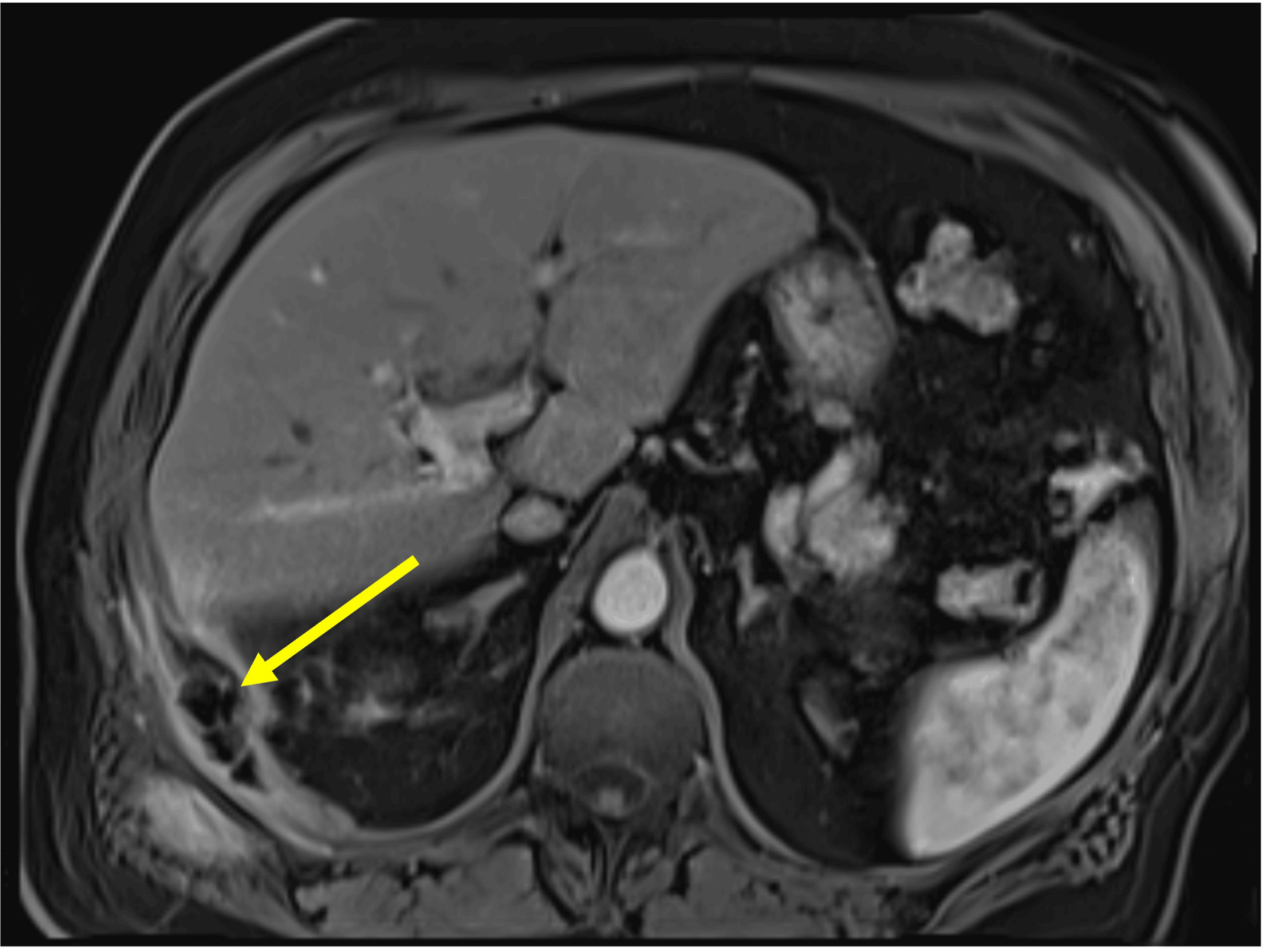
Magnetic resonance cholangiopancreatography (MRCP) findings 10 days after image-guided drainage of the perihepatic abscess. Axial T2-weighted HASTE sequence demonstrates multiple calculi (yellow arrow) up to 16 mm in size within the perihepatic abscess cavity.
